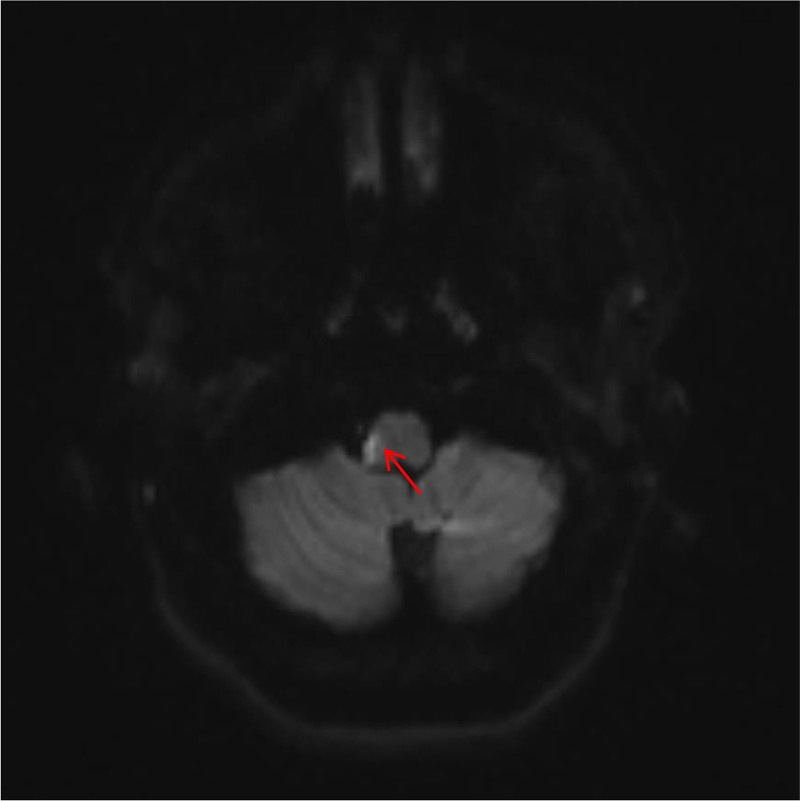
On January 25, 2019, the DWI sequence suggests a lacunar infarction in the medulla oblongata (arrows indicate anatomical location). DWI = diffusion weighted imaging.
